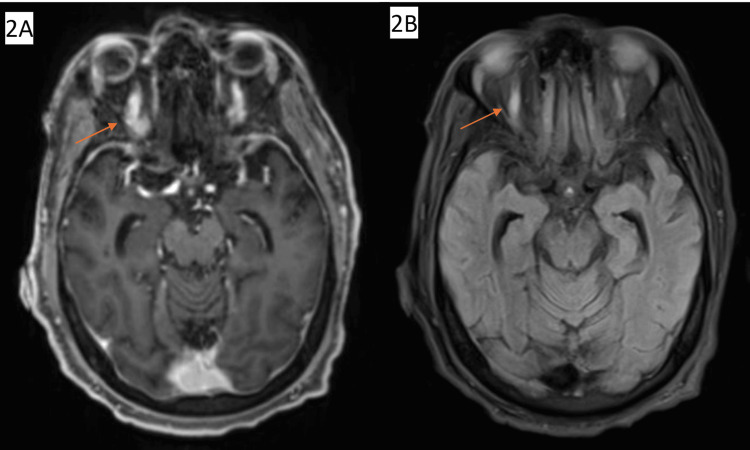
MRI Brain (A, B) in the axial plane showing right superior ophthalmic vein enlargement.
